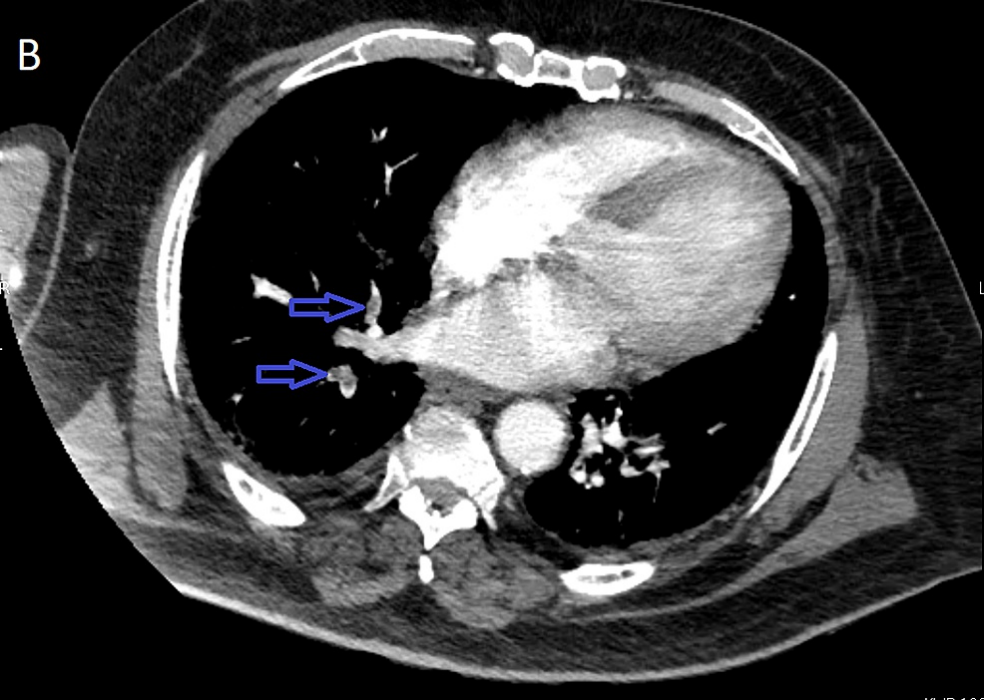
CT chest with contrast demonstrating filling defects in right lower lobe subsegmental (blue arrows) arteries suggestive of pulmonary embolism.
